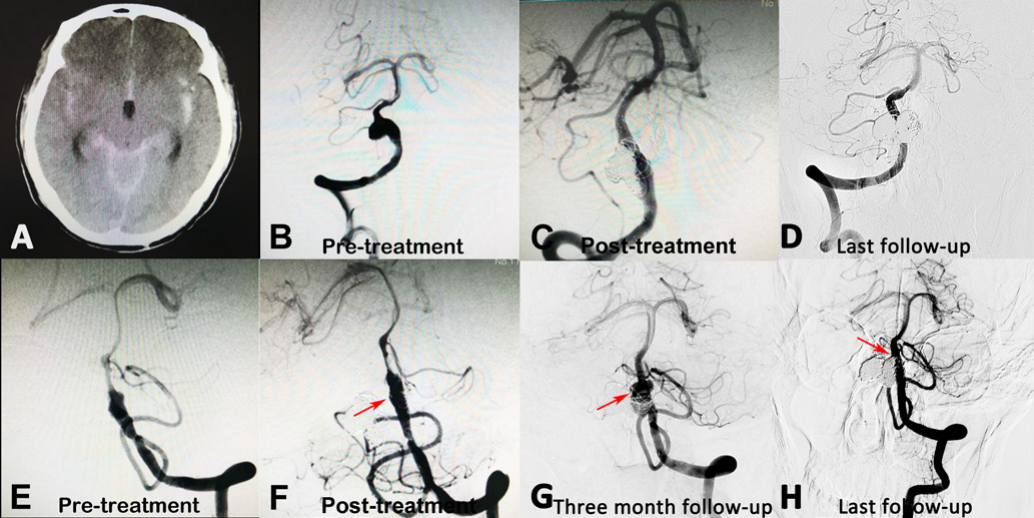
(A) A patient showing subarachnoid haemorrhage on CT imaging. (B and E) Digital subtraction angiography showed bilateral IVADAs. The ruptured side could not be determined based on the CT scan. (C and F) Thus, for this ruptured patient, both aneurysms were treated with stent-assisted coil embolisation at one stage, and a near-complete obliteration was achieved. (G) At the 3-month follow-up, the left IVADA recanalised, and the right IVADA was stable. The recanalised IVADA was further retreated with recoiling. (D and H) Both IVADAs were stable at the last follow-up angiography. IVADAs, intracranial vertebral artery dissecting aneurysms.
